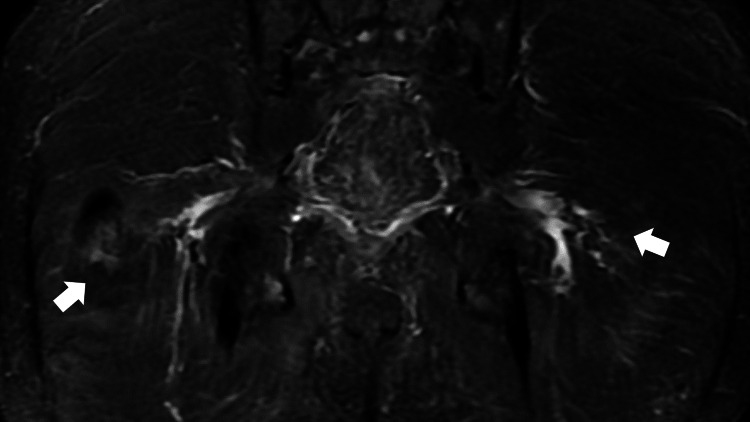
A pelvic magnetic resonance imaging scan (coronal plane) showing high signals in the bilateral sciatic nerves (arrows)
